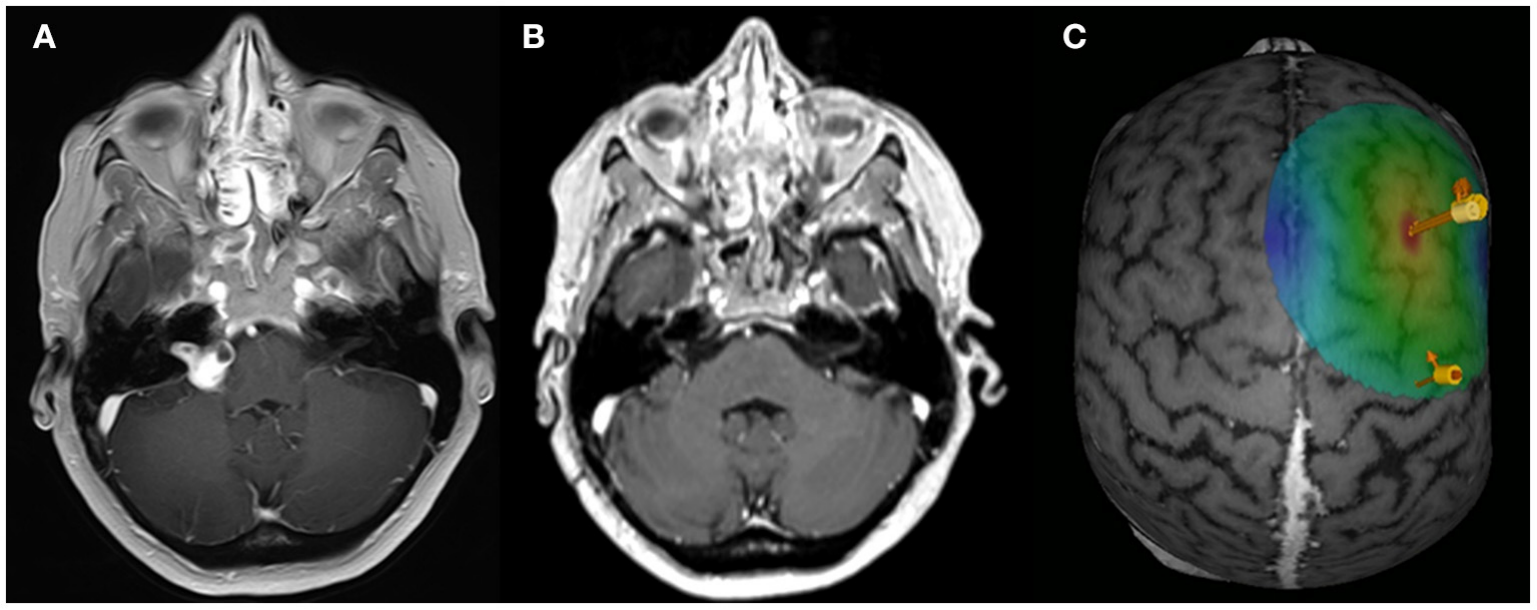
rTMS paradigm. Exemplary axial MRI data (contrast-enhanced T1 sequence) of patient ID1 with a right-sided VS for the preoperative (A) and post-operative (B) situation. (C) Exemplary data of the rTMS application site. Arrows are indicating the APB hotspot and the rTMS on the DLPFC.
